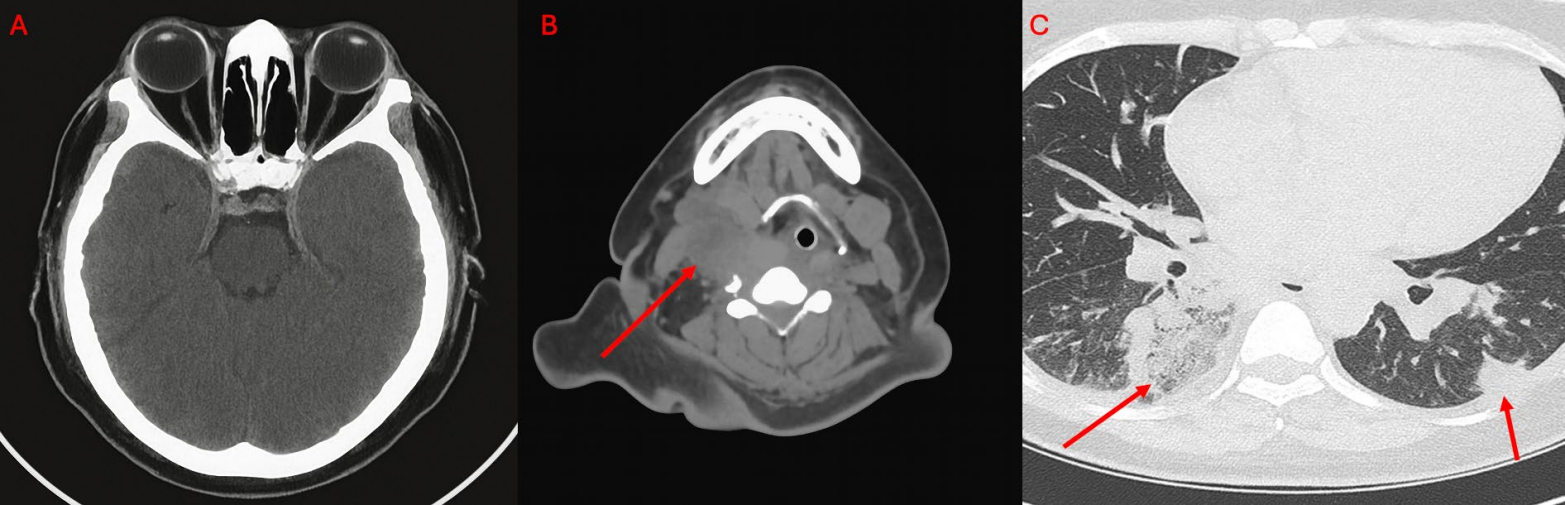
(A) Axial non‐contrast head computed tomography (CT) showing no evidence of intracranial extension, venous sinus thrombosis, or parenchymal abnormalities. (B) Axial contrast‐enhanced neck CT demonstrating a right‐sided hypodense deep neck collection with surrounding soft‐tissue edema and thrombosis of the right internal jugular vein, evidenced by non‐opacified intraluminal material (arrow). (C) Axial contrast‐enhanced chest CT demonstrating multiple peripheral cavitary nodules and consolidations, predominantly in the right lower lobe, consistent with septic pulmonary emboli (arrows).
